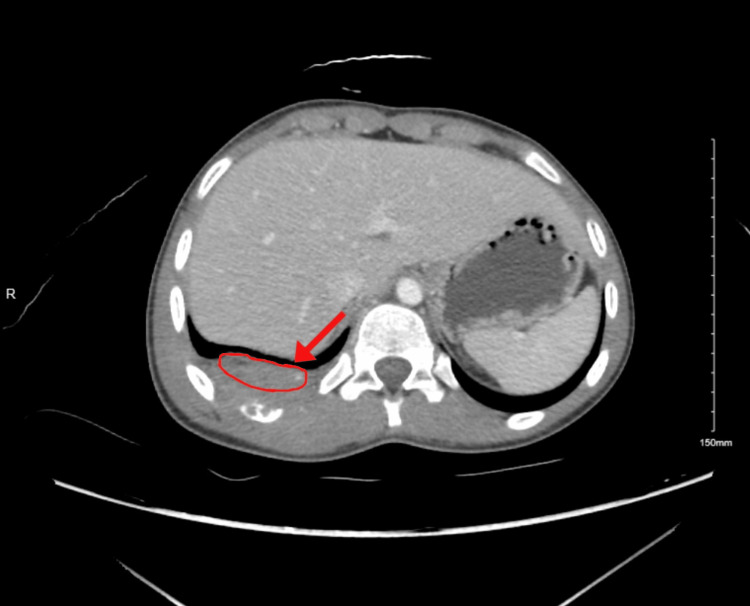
Abdominal CT of posterior chest wall mass consistent with Ewing’s sarcoma
